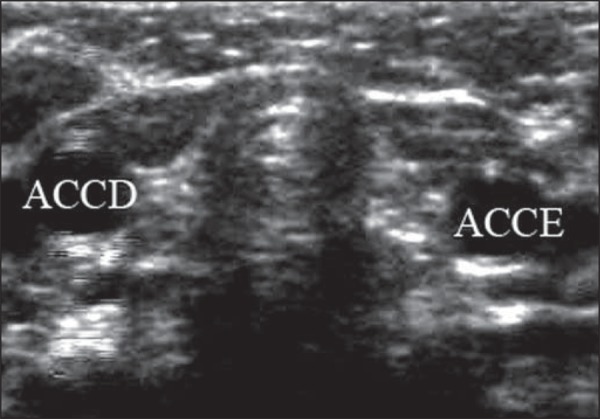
Ultrasonography of cervical region. In this child, the thyroid gland could not be
